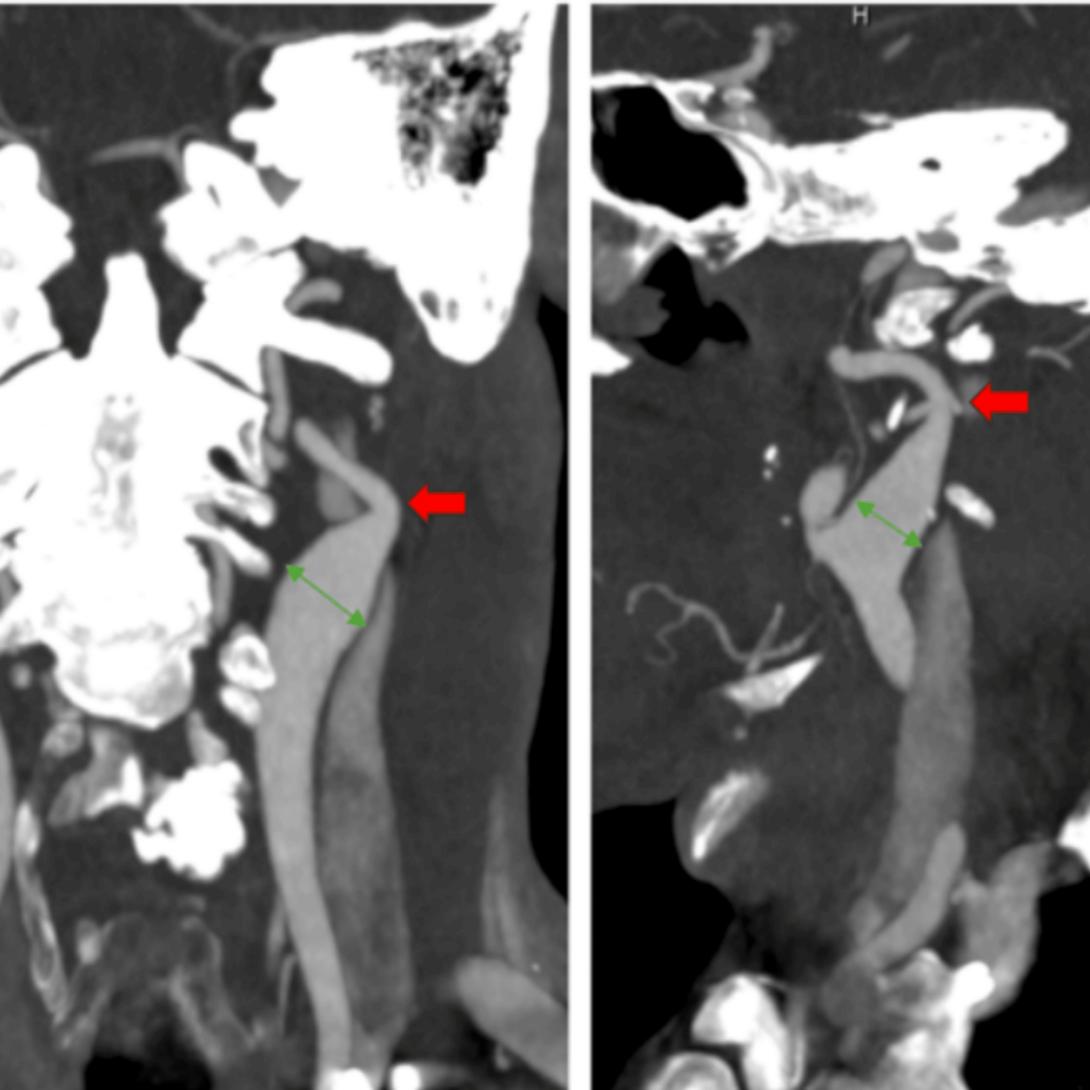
Computed tomography angiography (CTA) of the head and neck. CTA of the head and neck demonstrating tortuous carotid arteries, significant stenosis, and bilateral bulbar dilation; all features that may indicate underlying fibromuscular dysplasia. The red arrow demonstrates significant stenosis of the left carotid artery. The green arrow indicates significant carotid bulbar dilation.
